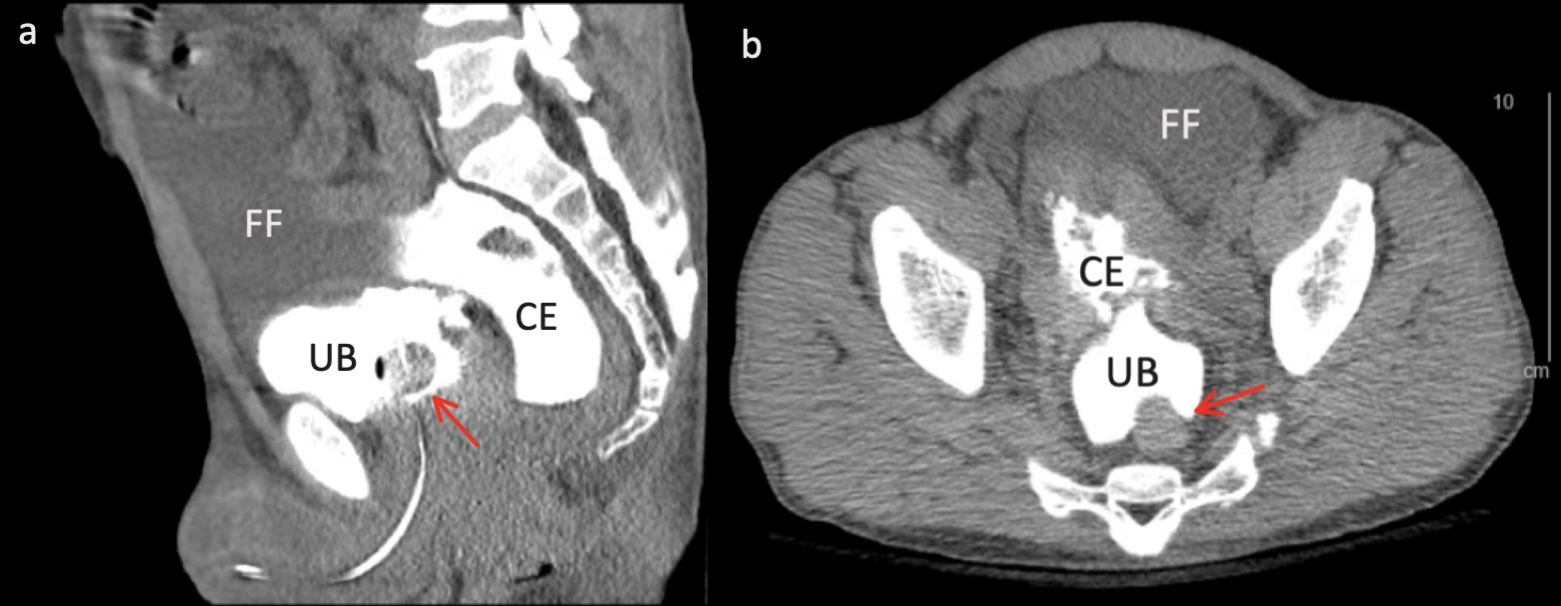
Computed tomography cystography demonstrating urinary contrast extravasation. In sagittal view (a), the Foley catheter appears filled with contrast. In both sagittal and axial (b) views, the catheter balloon (indicated by the red arrow) can be visualized within the urinary bladder (UB). Contrast extravasation (CE) can be seen emerging through the defect at the bladder dome and residing posteriorly in the supine patient. Eventually, the large amount of extravasated urinary contents has pushed the initial contents and free fluid (FF) into the anterior space as well.
